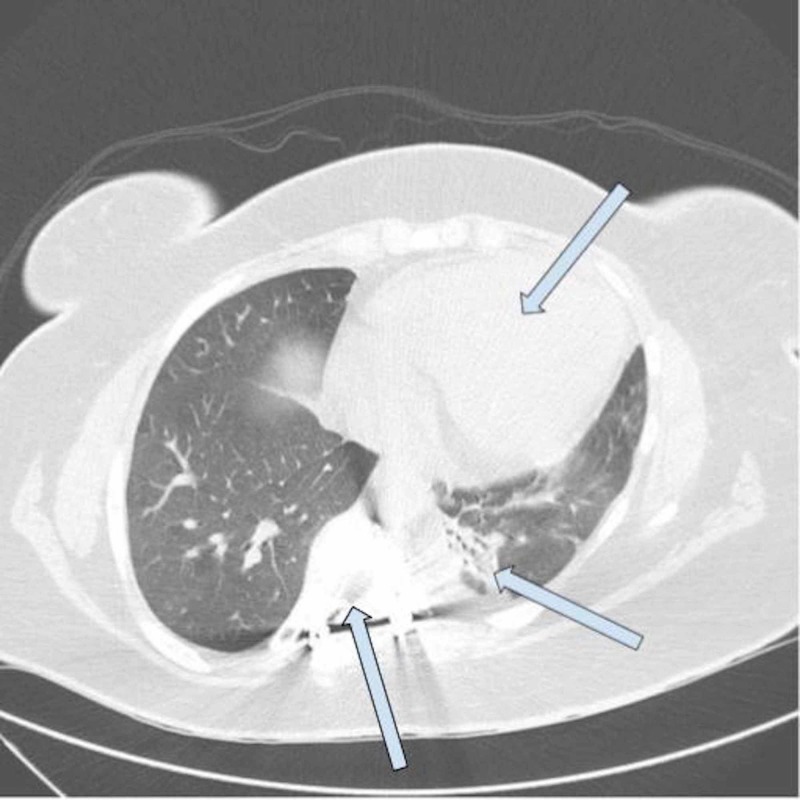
Computed tomography (CT) chest without contrast Scoliosis within the thoracic spine. Minimal atelectasis of left lower lobe. No pleural effusions. Moderate cardiomegaly with no pericardial effusion.
